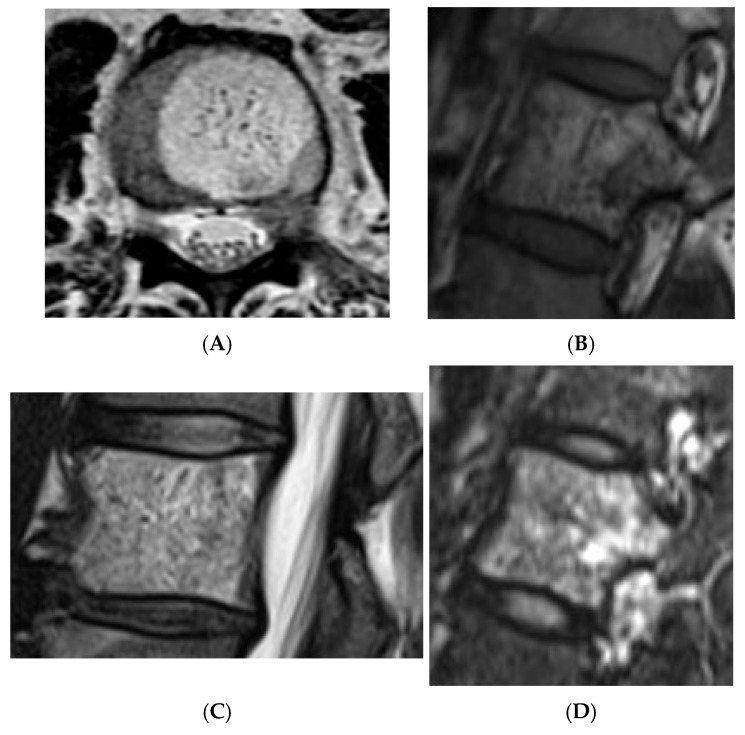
Typical haemangioma of the vertebral body. (A) T1W axial MRI. (B) T1W sagittal MRI. (C) T2W sagittal MRI. (D) STIR sagittal MRI.
